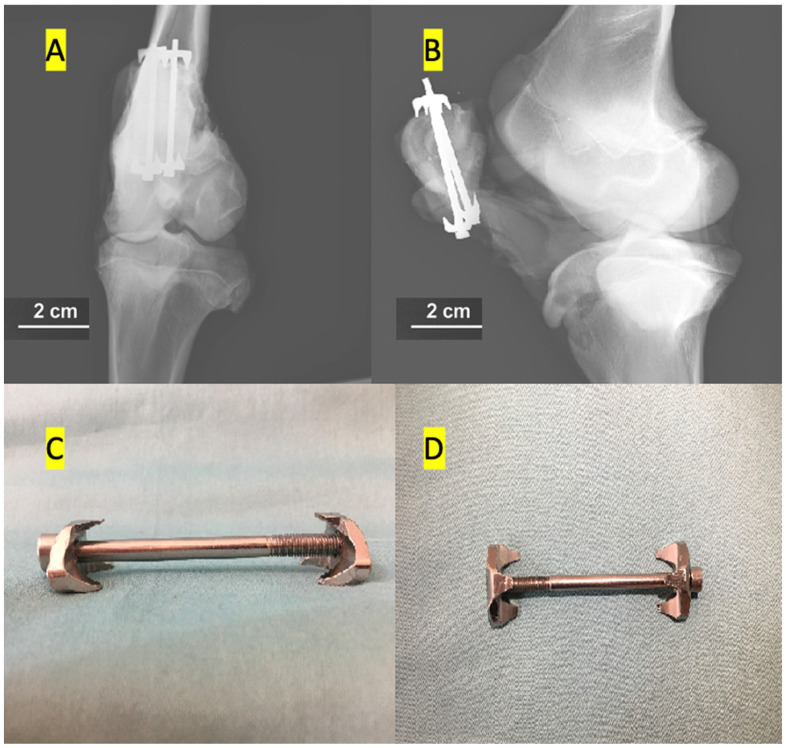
Radiographic and macroscopic views of the newly designed implant for patella fracture fixation. (A) Anteroposterior X-ray of the calf knee after fixation with the new-generation implant. (B) Lateral X-ray of the calf knee demonstrating the implant in situ. (C) Lateral view of the newly designed implant. (D) The top view of the implant shows a bilateral hook configuration.
